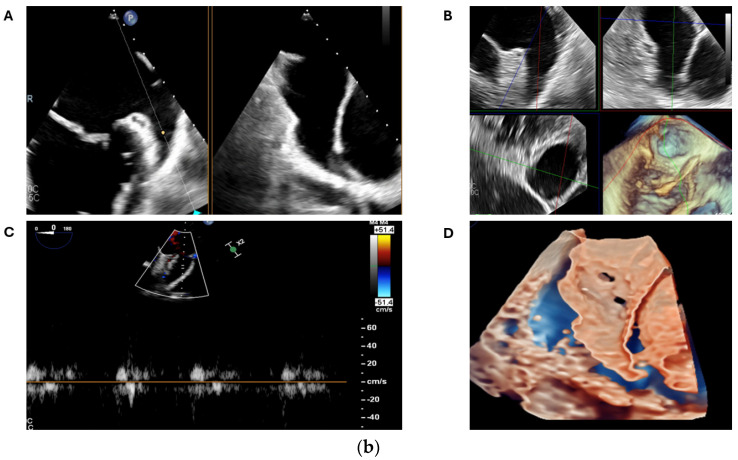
(a) A 70-year-old gentleman with atrial fibrillation on an appropriate dosage of warfarin was found to have a LAA thrombus on cardiac CT. Despite increasing the target INR range to 3-4, the filling defect persisted on repeat cardiac CTs (delayed phase shown here in images (A,B)), with a Hounsfield Unit of consistently < 50 HU. (b) The patient underwent a TOE to further assess the LAA for the presence of a persistent thrombus despite optimal anticoagulation. (A) X-plane 2D TOE showing a possible spontaneous echo contrast in the distal LAA, also seen on the 3D multiplanar reformat (B). (C) Pulsed-wave velocity assessment of the LAA showed a doppler velocity of 17 cm/s, in keeping with slow flow or spontaneous echo contrast. However, on the 3D glass view analysis (D), the entire appendage is clearly visualised with no evidence of thrombus, highlighting the importance of advanced 3D TOE as the gold standard imaging modality for the assessment of LAA thrombus. The patient underwent successful LAAO with no complications, and follow-up imaging showed no evidence of PDL or DRT. TOE: Transoesophageal echocardiogram; LAA: Left atrial appendage; LAAO: Left atrial appendage occlusion; 2D: Two-dimensional; 3D: Three-dimensional.
